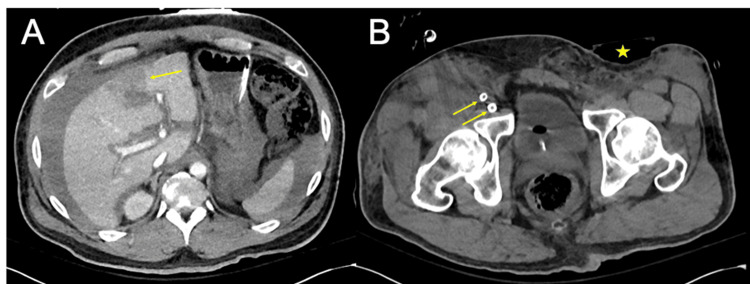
Arterial and delayed phase contrast-enhanced computed tomography (CT). Axial (A) CT scan in the arterial phase demonstrating a liver laceration involving segment IV (yellow arrow), without evidence of active contrast extravasation, and (B) delayed-phase contrast-enhanced CT demonstrating right common femoral artery and vein cannulation for ECMO support (yellow arrows), with a femoral compression (FemoStop; Temecula, CA: Abbott) device (yellow star) over the left groin. ECMO: extracorporeal membrane oxygenation
